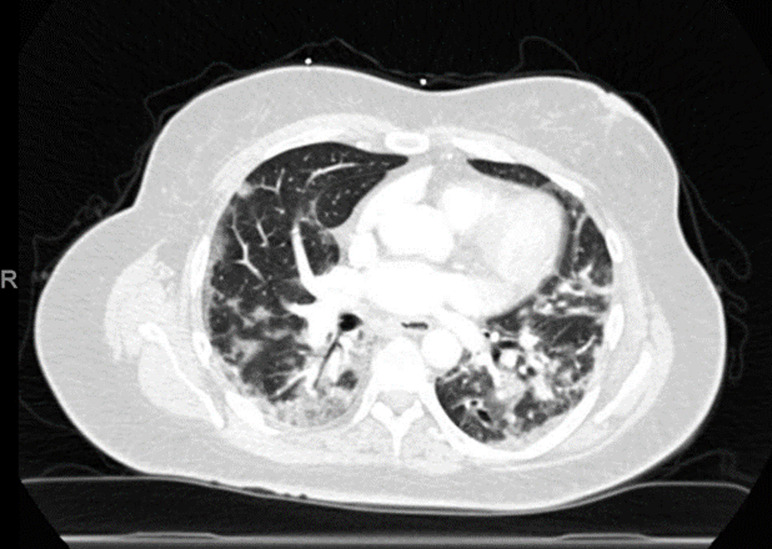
chest computed tomography of patient with COVID-19 pneumonia showing bilateral multifocal opacities in lungs
